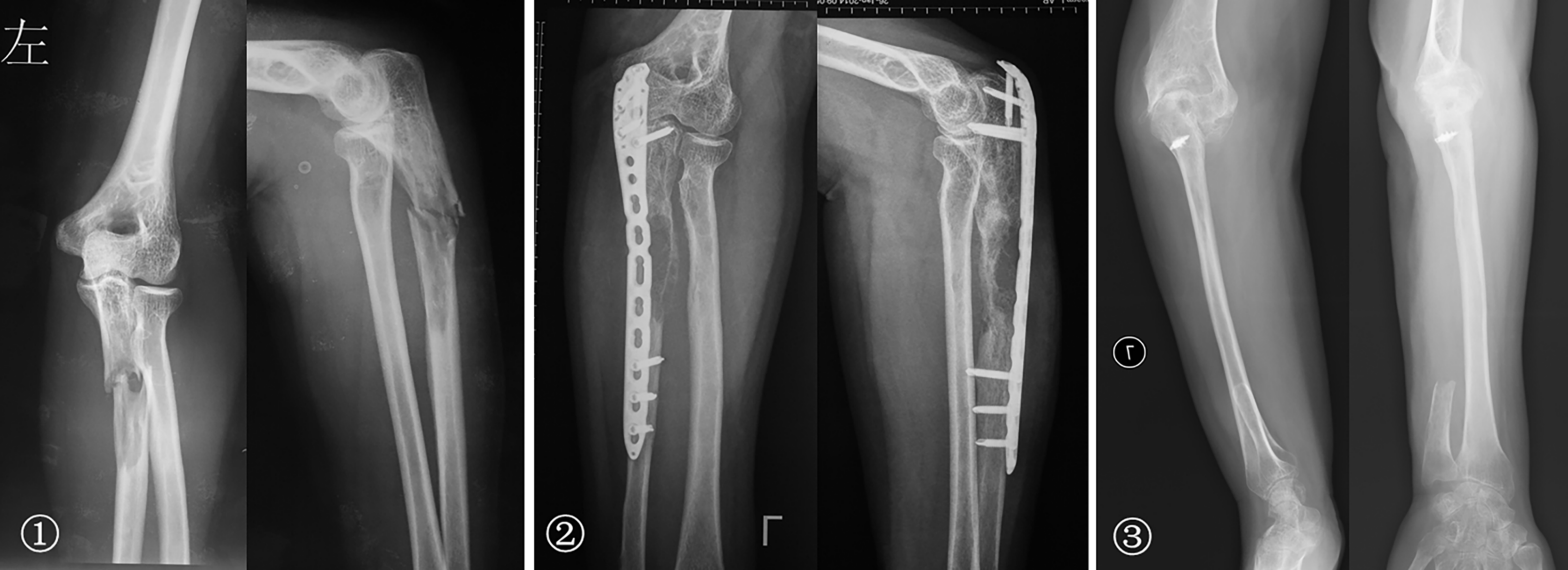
① The preoperative radiographs of a 51-year-old woman with osteosarcoma of the proximal ulna. ② An unplanned excision and tumor recurrence occurred. ③ The radial head was displaced and inserted into the intercondylar of the humerus after proximal ulna resection; the rotation function of the forearm is shown 76 months postoperatively.
